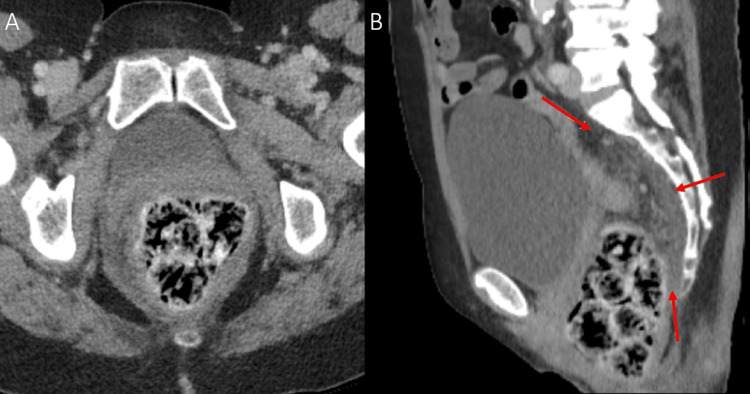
Axial (A) and sagittal (B) contrast-enhanced computed tomography (CT) of the lower pelvis. The rectum demonstrates a large rectal stool burden, mild rectal wall thickening, and peri-rectal and presacral edema and fat stranding (red arrows). On the sagittal view, the urinary bladder is distended.
